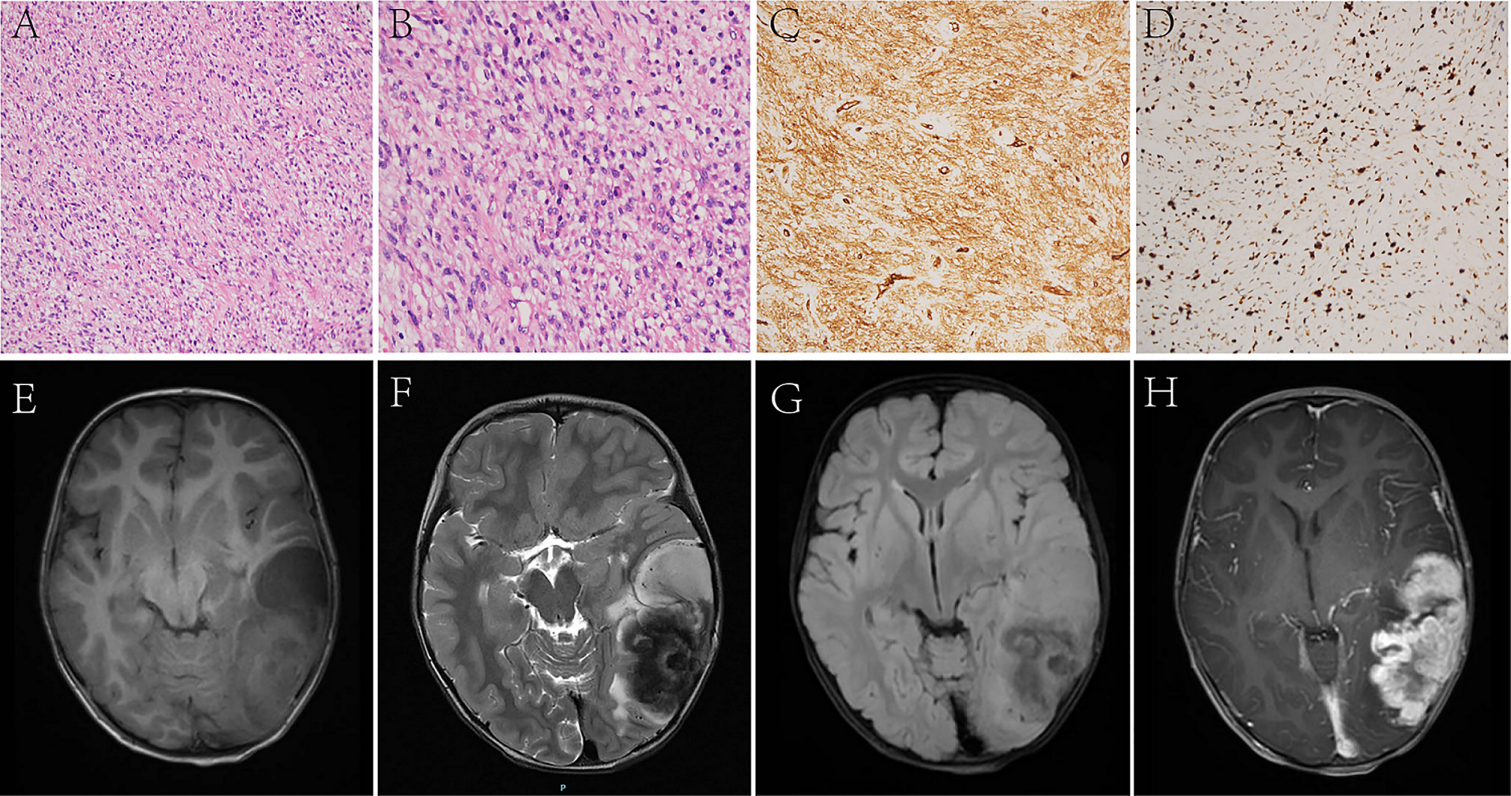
In Case 2, the tumor cells are long spindles, relatively uniform in size, and arranged in bundles (A, B). The IHC analysis displays positiveness for CD34 (C), and the Ki-67 LI is about 30% (D). MRI shows that the tumor is located in the left temporo-occipital lobe, a solid-cystic irregular appearance, T1WI (E), T2WI (F), and T2 FLAIR (G) shows heterogeneous intensity, contrast-enhanced T1WI (H) shows garland-like enhancement.
